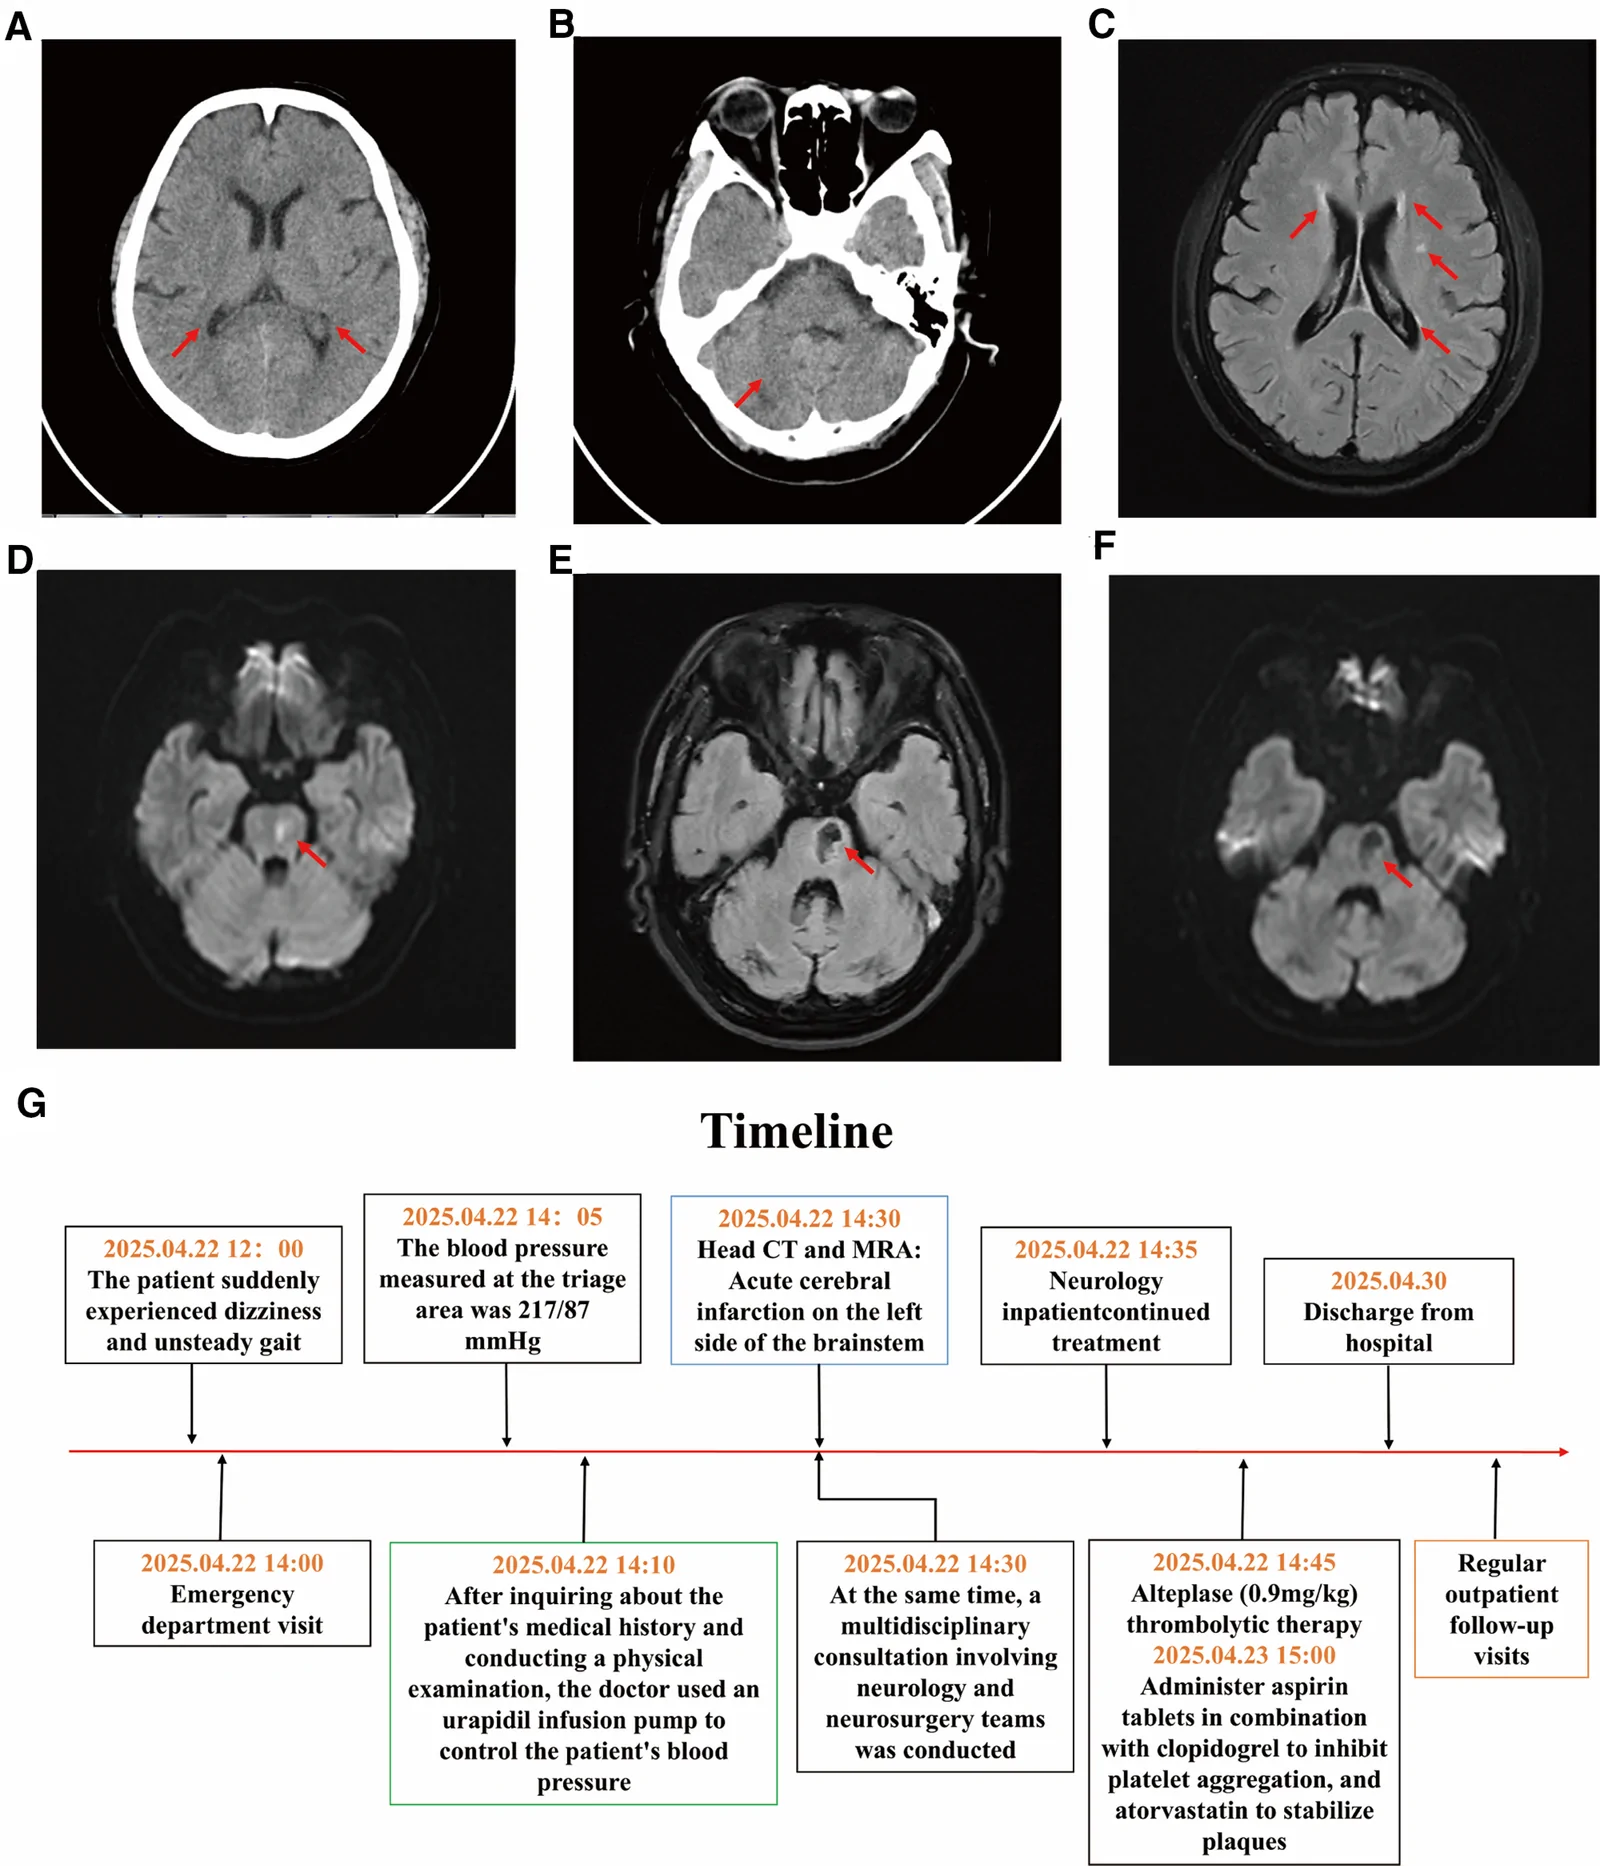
Cranial imaging of the patient. (A, B) CT scans demonstrate patchy hypodense regions in the right cerebellum and bilateral periventricular white matter, with poorly defined margins. (C, D) MRA images: small patchy hyperintense signals on DWI in the left brainstem; other sequences are inconclusive. Multiple small spot-like abnormal signals are observed in the bilateral centrum semiovale and periventricular white matter, with poorly defined borders, slightly hypointense on T1WI, hyperintense on T2WI/FLAIR, and no obvious signal change on DWI. (E, F) Follow-up images show multiple small spot-like abnormal signals in the left brainstem and bilateral centrum semiovale/periventricular white matter, with poorly defined borders, slightly hypointense on T1WI, and hyperintense on T2WI/FLAIR; patchy hypointense signals are seen in the left brainstem on DWI without obvious hyperintensity. (G) Timeline of events reported in this case. CT = computed tomography, DWI = diffusion-weighted imaging.
